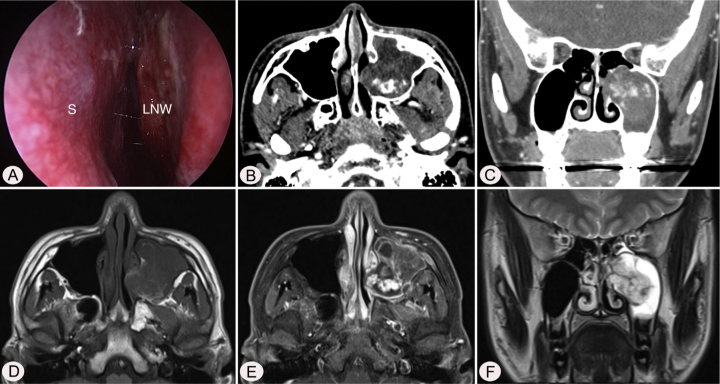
Preoperative endoscopic and radiologic findings. Nasal endoscopy (A) shows bulging of left Lateral Nasal Wall (LNW). Axial (B) and coronal (B) computed tomographic images shows heterogenous enhancing mass in left maxillary sinus without adjacent bony involvement. The mass shows mixed high signal intensity on T1 axial (D), avid enhancement on postcontrast T1 axial (E), and heterogenous high signal intensity on T2 coronal (F) magnetic resonance images. S, nasal septum.
